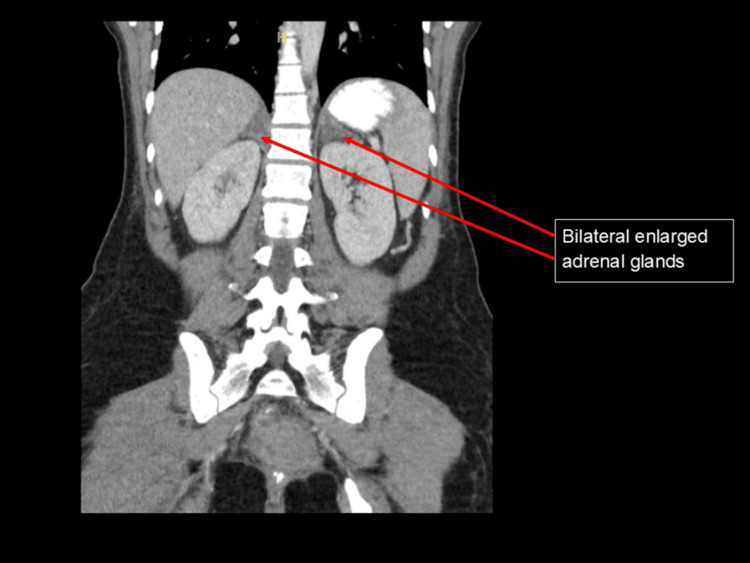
CT of the abdomen with and without contrast. Coronal view showing marked bilateral adrenal gland thickening.
